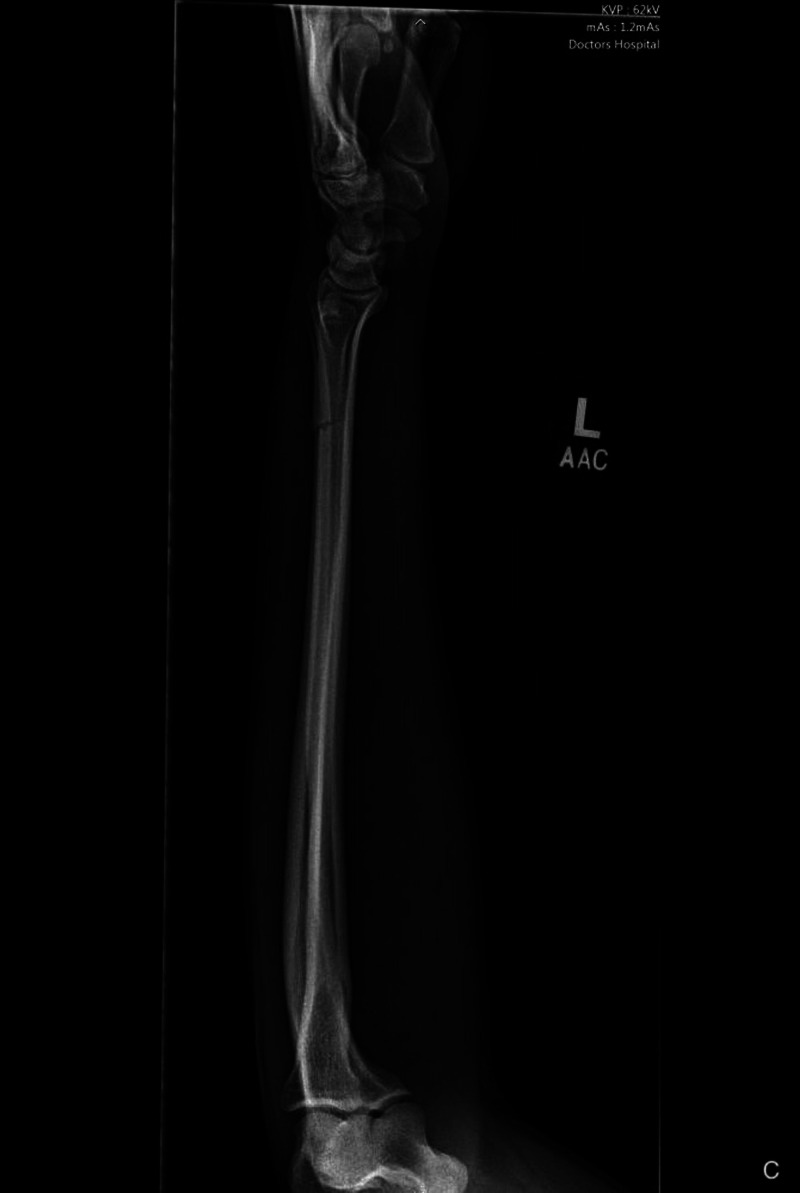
Lateral injury film/radiograph of the left forearm demonstrating an isolated ulnar shaft fracture with minimal displacement and angulation.
